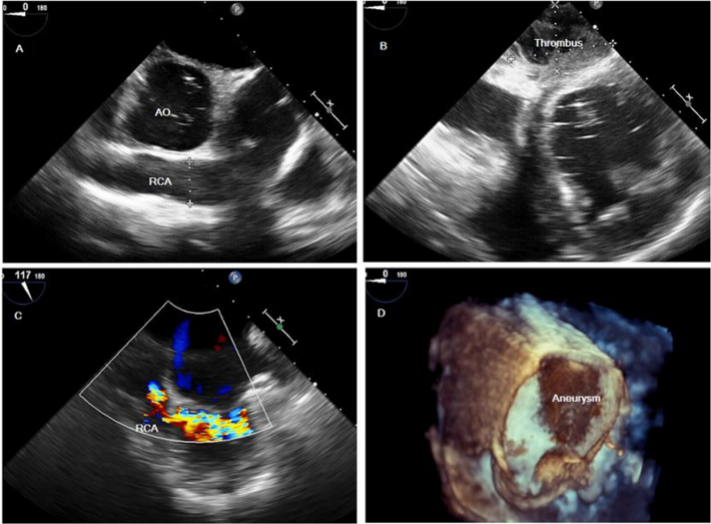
Real-time three-dimensional echocardiography (a) The dilated RCA (b) Thrombus in the aneurysm, which measured 4.4 × 2.8 cm (c) Extremely tortuous RCA draining into the LV through the inferoposterior wall of the LV (d) Three-dimensional image of the aneurysm
